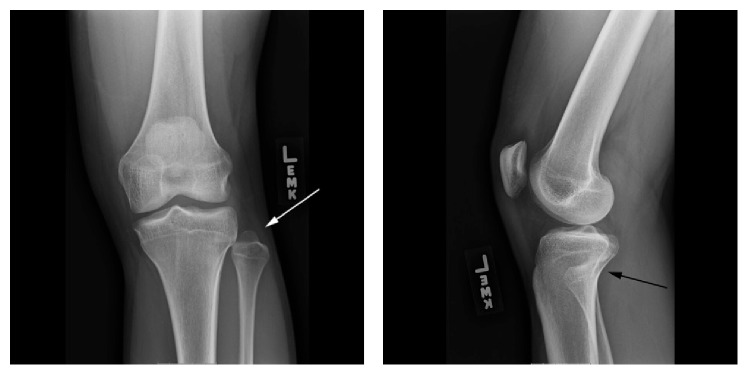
AP and lateral X-ray views of the left knee showing Type II anterolateral tibiofibular dislocation.
